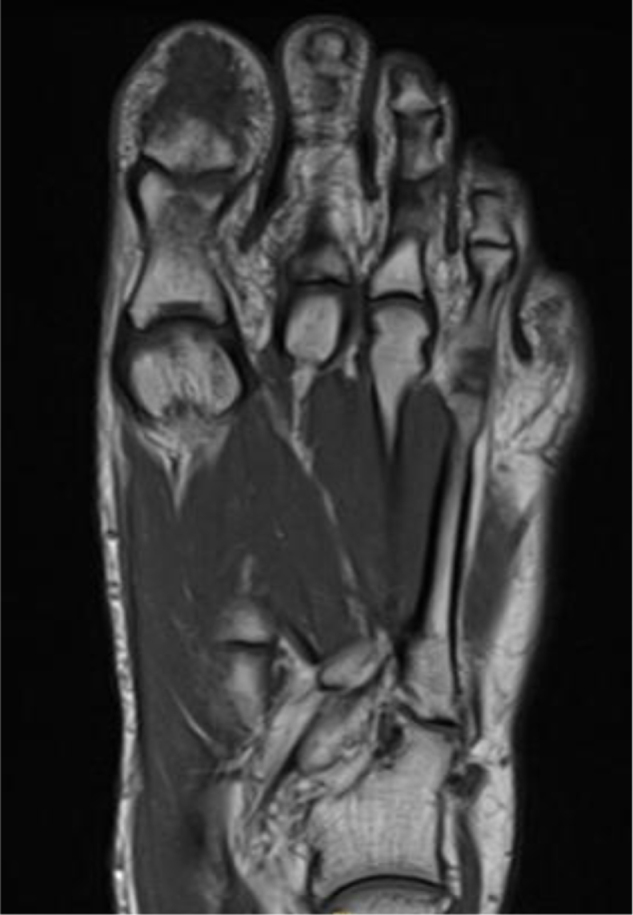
Coronal MRI of the left foot demonstrates a heterogeneously enhancing mass centered around the distal phalanx of the first toe.
